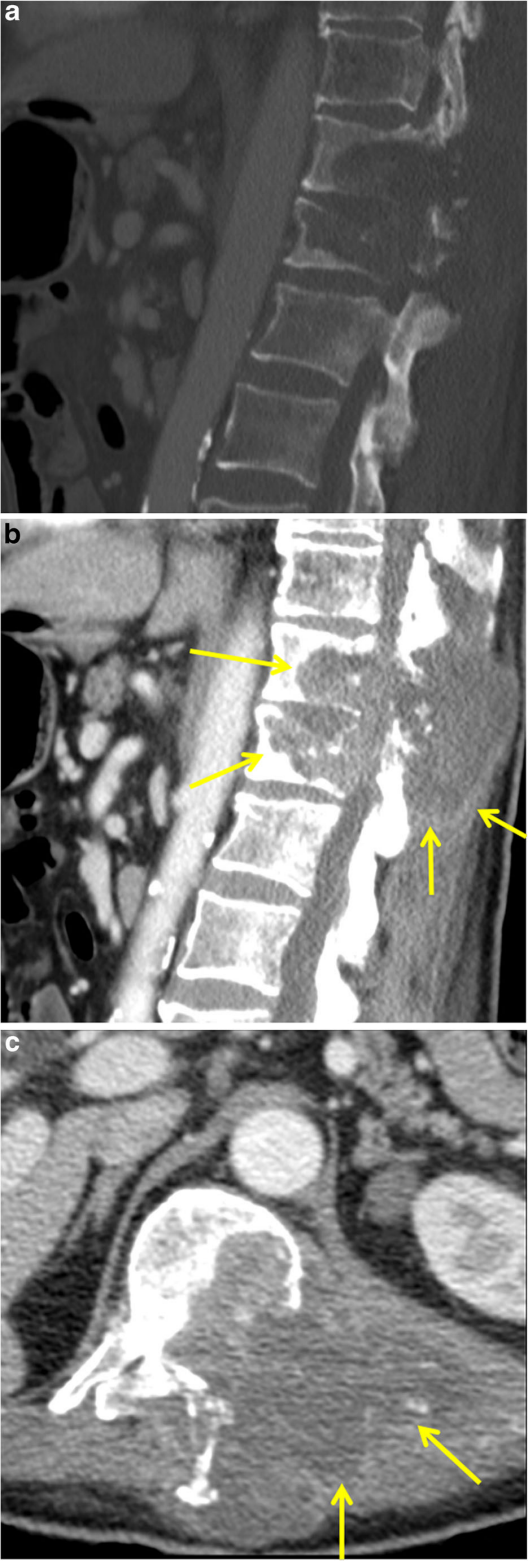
Malignant spinal cord compression of the thoracic spine in a 56-year-old man with stage IV non-small cell lung cancer. Sagittal enhanced CT image in bone (a) and soft-tissue (b) window setting at the thoracolumbar level shows confluent lytic bone lesions in the T11 and T12 vertebrae with disruption of the posterior wall and extending into the posterior elements. Also, note the large soft-tissue mass (*yellow arrows*) extending in the spinal canal resulting in a compression of the thoracic spinal cord. Axial enhanced CT image in soft tissue window (c) clearly shows the extension of the soft tissue mass in the spinal canal, while the spinal cord is no longer discernible
